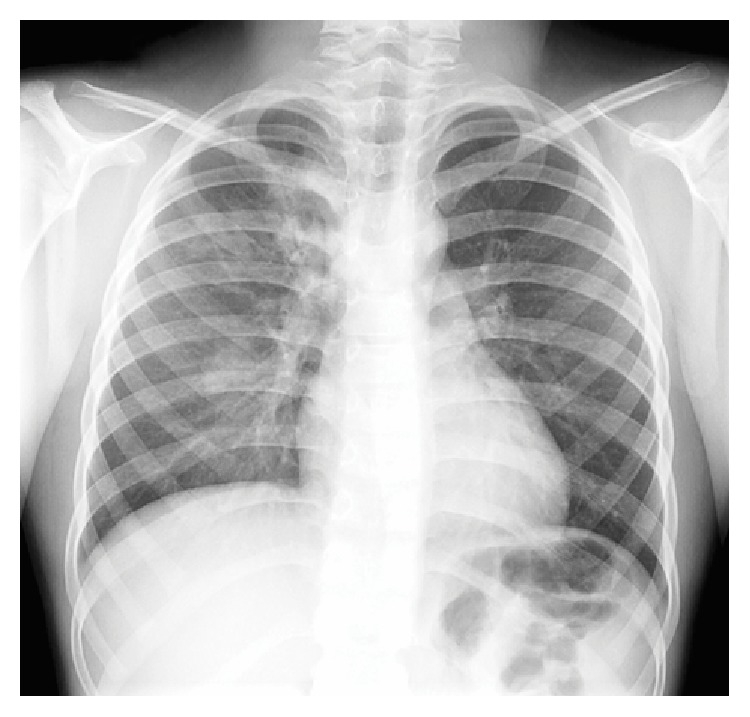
Initial chest X-ray: right upper and lower lobe infiltrations.
